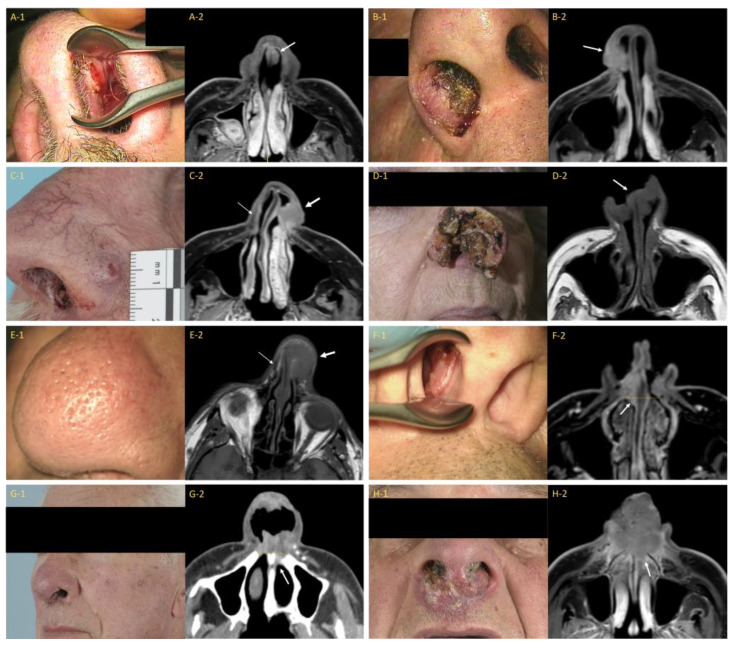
Clinical photographs and corresponding imaging studies of patients with a carcinoma of the nasal vestibule for each stage of the Bussu et al. classification. (A-1) = Patient with a left-sided T1 carcinoma of the nasal vestibule (CNV); (A-2) = Corresponding axial postcontrast T1-weighted MR image with fat saturation (T1 MR Gd FS) showing subtle soft tissue thickening on the left side of the nasal septum (white arrow); (B-1) = Patient with a right-sided T2a CNV (caudal ala); (B-2) = Corresponding axial T1 MR Gd FS image displaying the tumor invading the cutis (white arrow); (C-1) = Patient with a left-sided T2b CNV with extension through the alar cartilage to the external skin of the nose; (C-2) = Corresponding axial T1 MR Gd FS image showing cartilage invasion and an irregular skin contour (thick white arrow). Compare to the contralateral side where the normal (low signal intensity) alar cartilage is visible (thin white arrow); (D-1) = Patient with a T2b CNV with extensive tissue destruction; (D-2) = Corresponding axial T1 MR Gd FS image showing the (bilateral) tumor (white arrow); (E-1) = Patient with a left-sided T2b CNV with peau d’orange suggesting the invasion of the (sub)cutis; (E-2) = Corresponding axial T1 MR Gd FS image. Note: absence of the alar cartilage (thick white arrow). Normal (low signal intensity) alar cartilage is present on the contralateral side (thin white arrow); (F-1) = Patient with a right-sided T3 CNV; (F-2) = Corresponding axial T1 MR Gd FS image shows tumor extension (white arrow) beyond the plane of the pyriform aperture (yellow line); (G-1) = Patient with a left-sided T3 CNV; (G-2) = Corresponding axial postcontrast CT image showing extension (white arrow) beyond the plane of the pyriform aperture (yellow line); (H-1) = Patient with a T4a CNV; (H-2) = Corresponding axial T1 MR Gd FS image displaying extension of the tumor into the anterior maxilla (white arrow).
